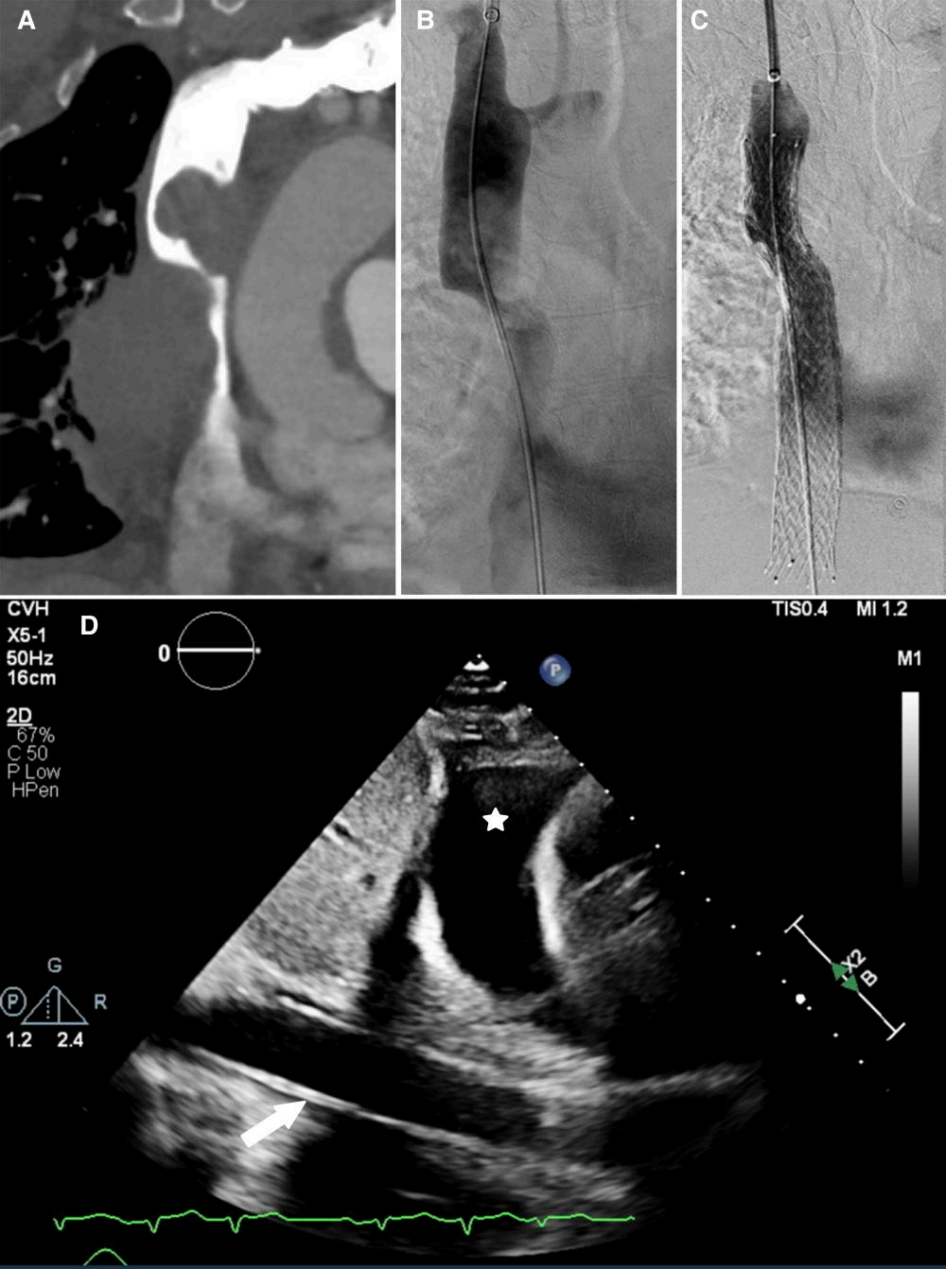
(*A*) Male in his 50 s (patient 1) with SVC syndrome related to squamous cell lung carcinoma. CT chest, coronal image, shows stenosis of the superior cavo-atrial junction due to the primary lung mass and mediastinal lymphadenopathy. (*B*) Superior vena cavagram shows severe stenosis of the superior cavo-atrial junction and upper right atrium due to extrinsic compression. (*C*) venogram post stent deployment shows relief of SVC stenosis with flow through the SVC-to-IVC bridging stent to the right atrium. (*D*) Transthoracic echocardiogram, subcostal view, identifies the IVC stent traversing the cavo-atrial junction (white arrow). A small pericardial effusion (white star) was unchanged from prior to the procedure and deemed too small to cause tamponade.
